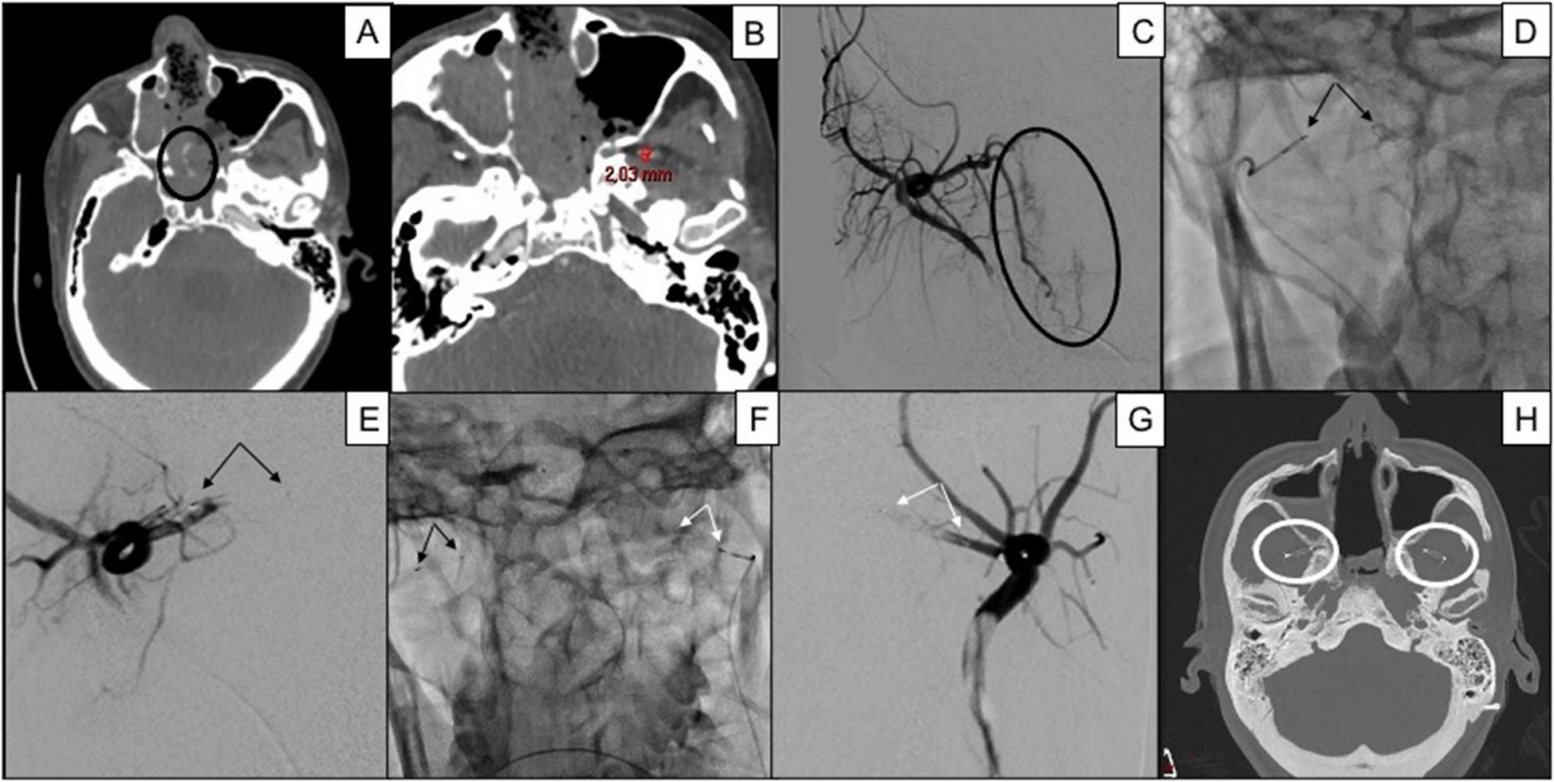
37 years old male affected by bleeding nasopharyngeal carcinoma after radiotherapy; bilateral internal maxillary artery embolization was performed, first with 300–500 microparticles and then, because of continuous bleeding, two MVP-5. In A, a contrast-enhanced CT scan in arterial phase shows active bleeding (black circle); in B, the target vessel internal maxillary artery is measured with caliper 2.03 mm, embolization being performed bilaterally; in C, right internal maxillary DSA confirms active bleeding (black circle); in D, MVP-5 is released through a 2.7 Fr microcatheter into the distal segment of the right internal maxillary artery, black arrows indicating distal and proximal radiopaque markers: in E, DSA confirms proper right internal maxillary artery occlusion immediately after MVP release; in F, a second MVP-5 (white arrows) is similarly positioned into the left internal maxillary artery (black arrows indicating MVP-5 previously released); in G, DSA confirms proper left internal maxillary artery occlusion immediately after MVP release; in H, an axial CT scan of the skull shows the two MVP-5 (white circle) not creating any metallic artifact
